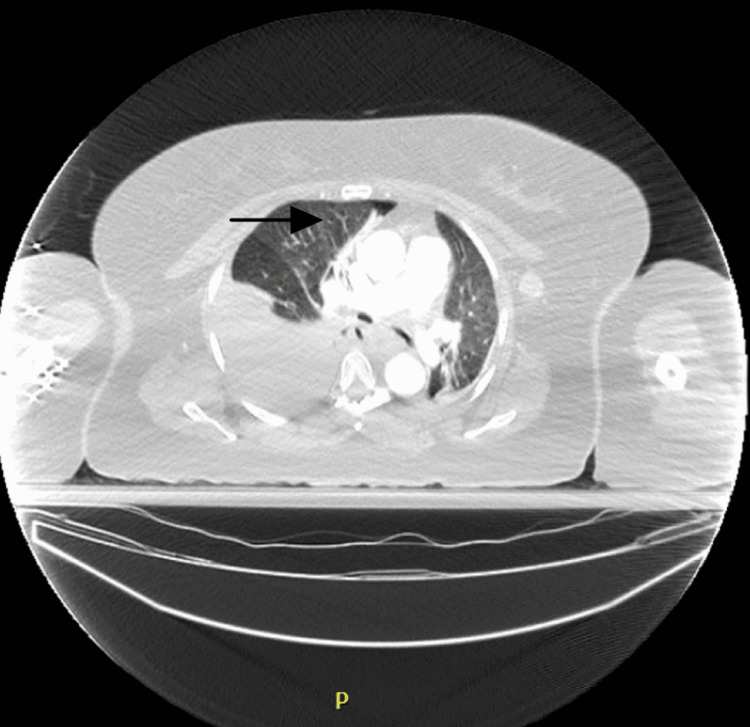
Computed tomographic angiogram of chest with IV contrast The image is again showing the large right-sided hemothorax with a significant mass effect on the right upper lobe and the mediastinal shift to the left.
